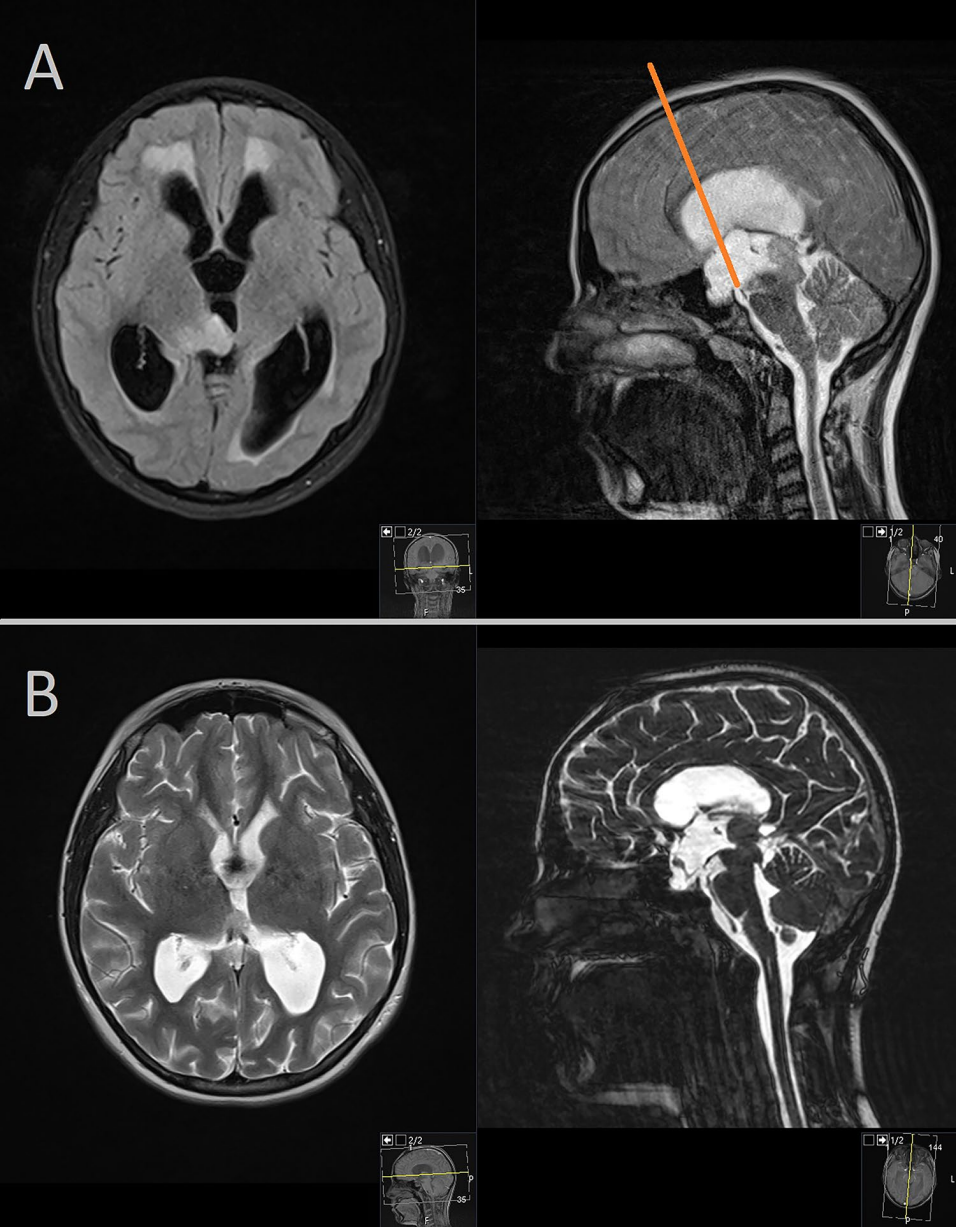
A MRI imaging revealing tumorous mass within the right thalamus and third ventricle. B Additional oncological treatment and follow-up MRIs performed postoperatively
